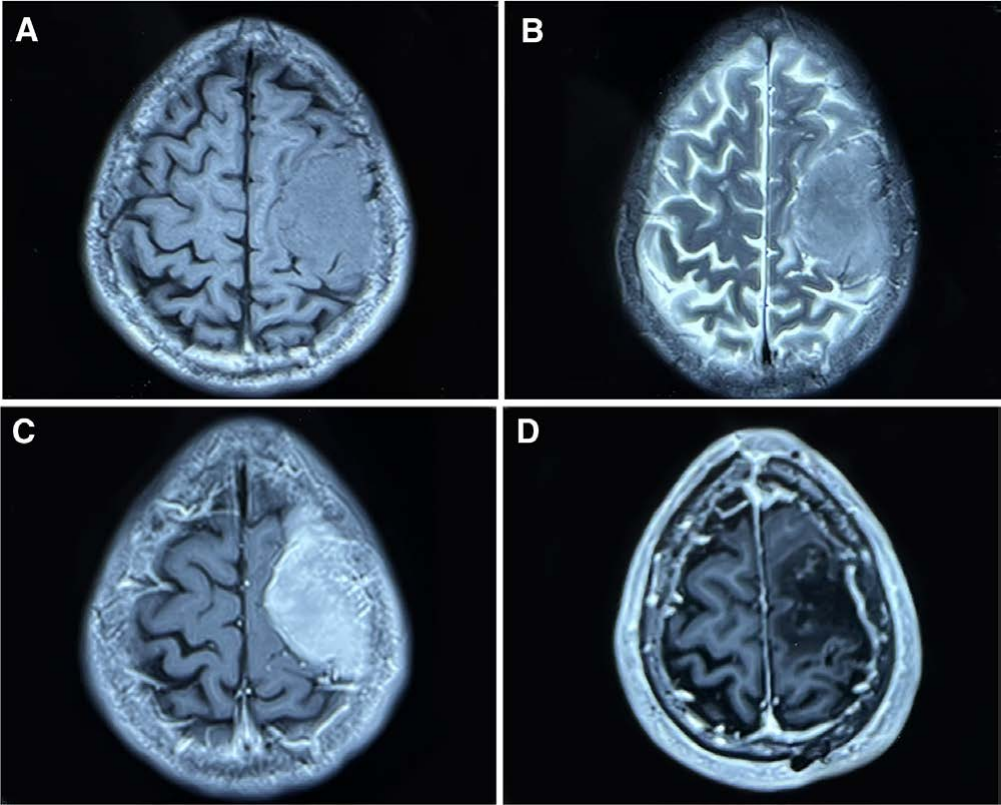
Preoperative MRI scans of the meningioma: *T*1-weighted, *T*2-weighted and *T*1-weighted with contrast enhancement (a, b and c, 7/18/2019); Postoperative MRI scans of the meningioma: *T*1-weighted with contrast enhancement (d, 12/26/2019), MRI = magnetic resonance imaging.
